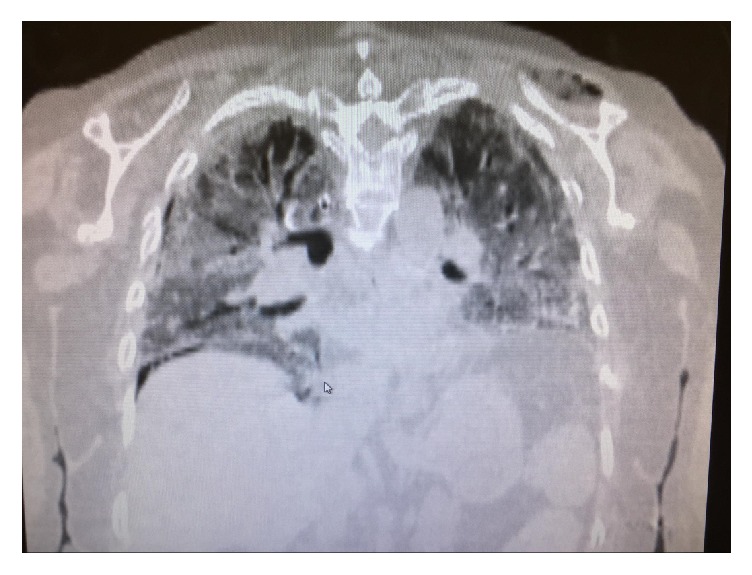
CT of chest showing bilateral infiltrates.
